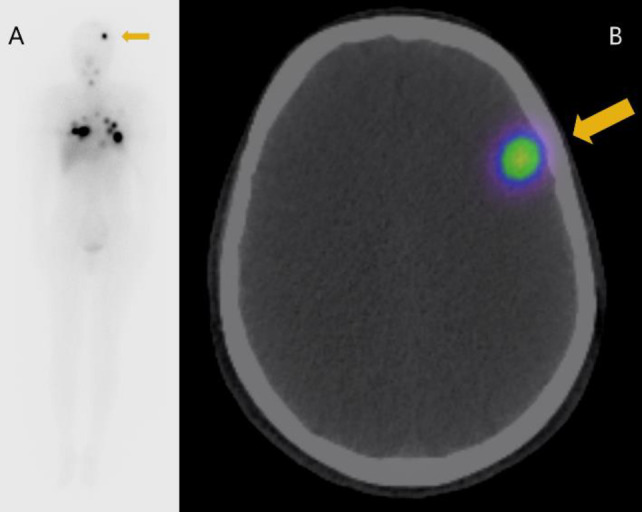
I-131 scintigraphy (A) and SPECT/CT (B) after the 1st radioactive iodine therapy (RAIT) showed accumulation in the left frontal lobe
